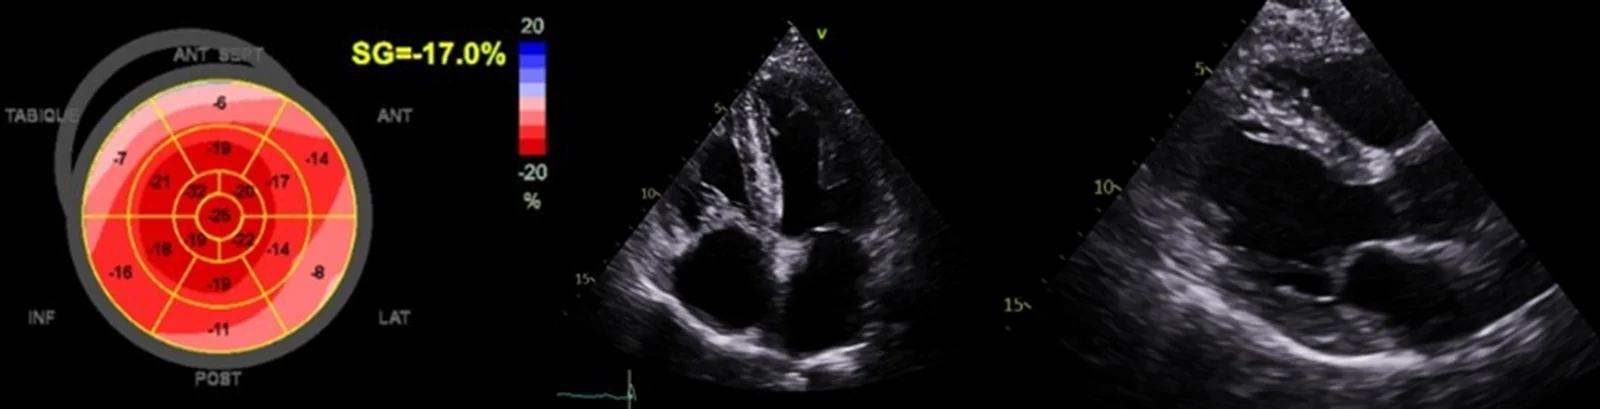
Transthoracic Echocardiography and Strain Imaging Apical 4-chamber and parasternal long-axis views demonstrating mild concentric left ventricular hypertrophy and preserved systolic function, with global longitudinal strain showing relative apical sparing.
